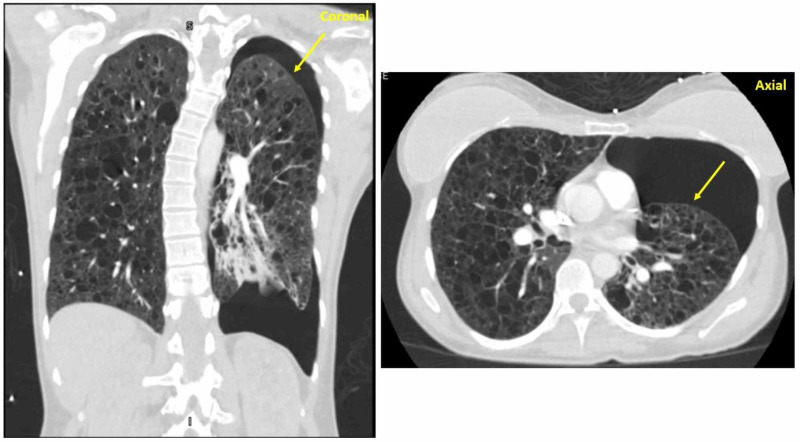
CT chest with IV contrast Coronal and axial views of left-sided pneumothorax shown with severe emphysema CT: computed tomography; IV: intravenous
